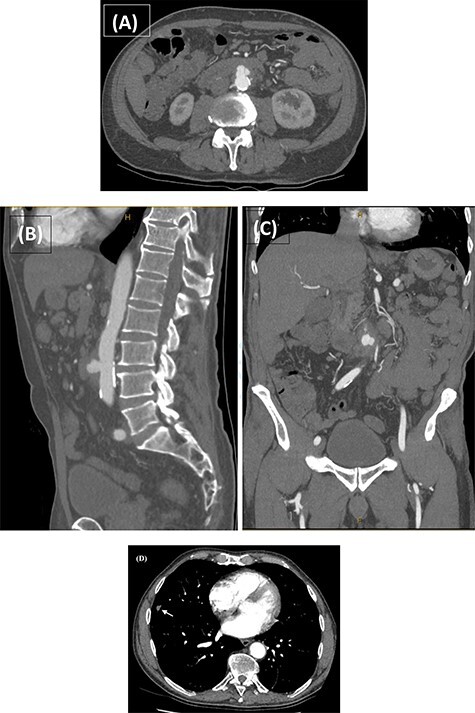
CTA performed following the MRCP demonstrating the presence of a thick-walled multi-lobulated saccular aneurysm arising from the anterior wall of the infrarenal abdominal aorta approximately at the level of the IMA origin. The aneurysm measures ~35 × 23 × 32 mm with adjacent soft tissue stranding and enlarged nodes in the aortocaval and left para-aortic region (A–C). The incidentally noted right middle lobe nodule is also demonstrated (D; white arrow).
